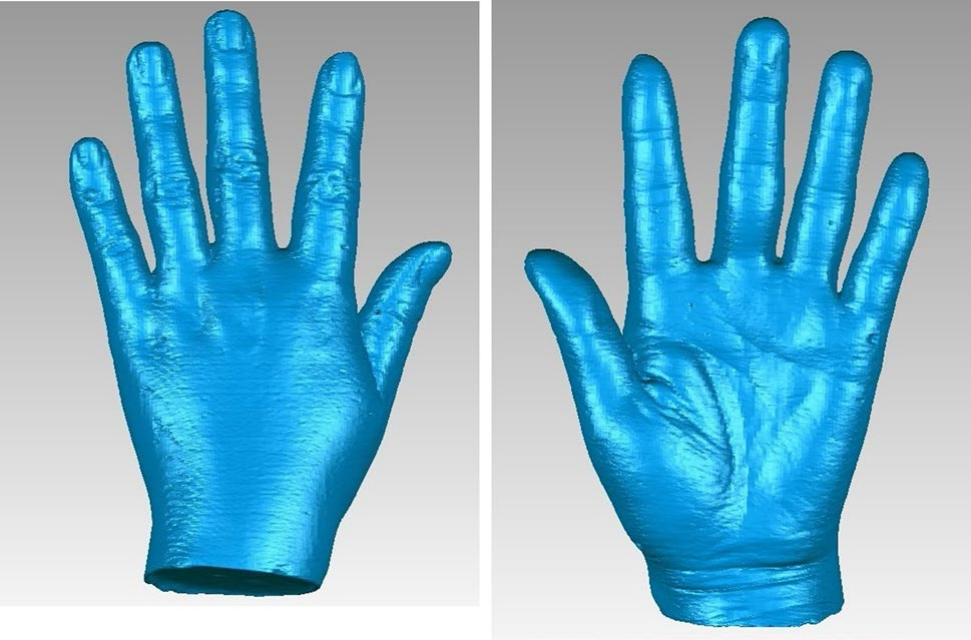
3D photographs of plaster hand model by CT scanning.
